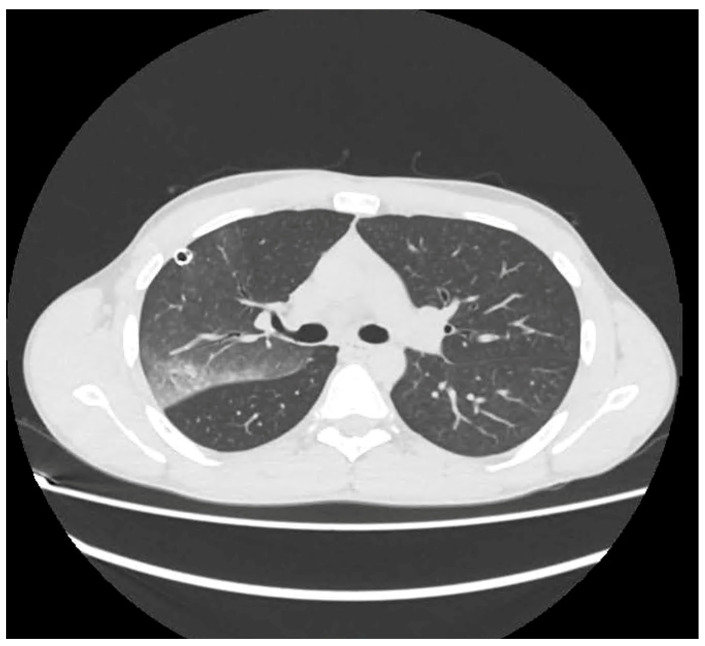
CT scan of right re-expansion pulmonary edema with alveolar filling and a consolidative area with air bronchogram.
